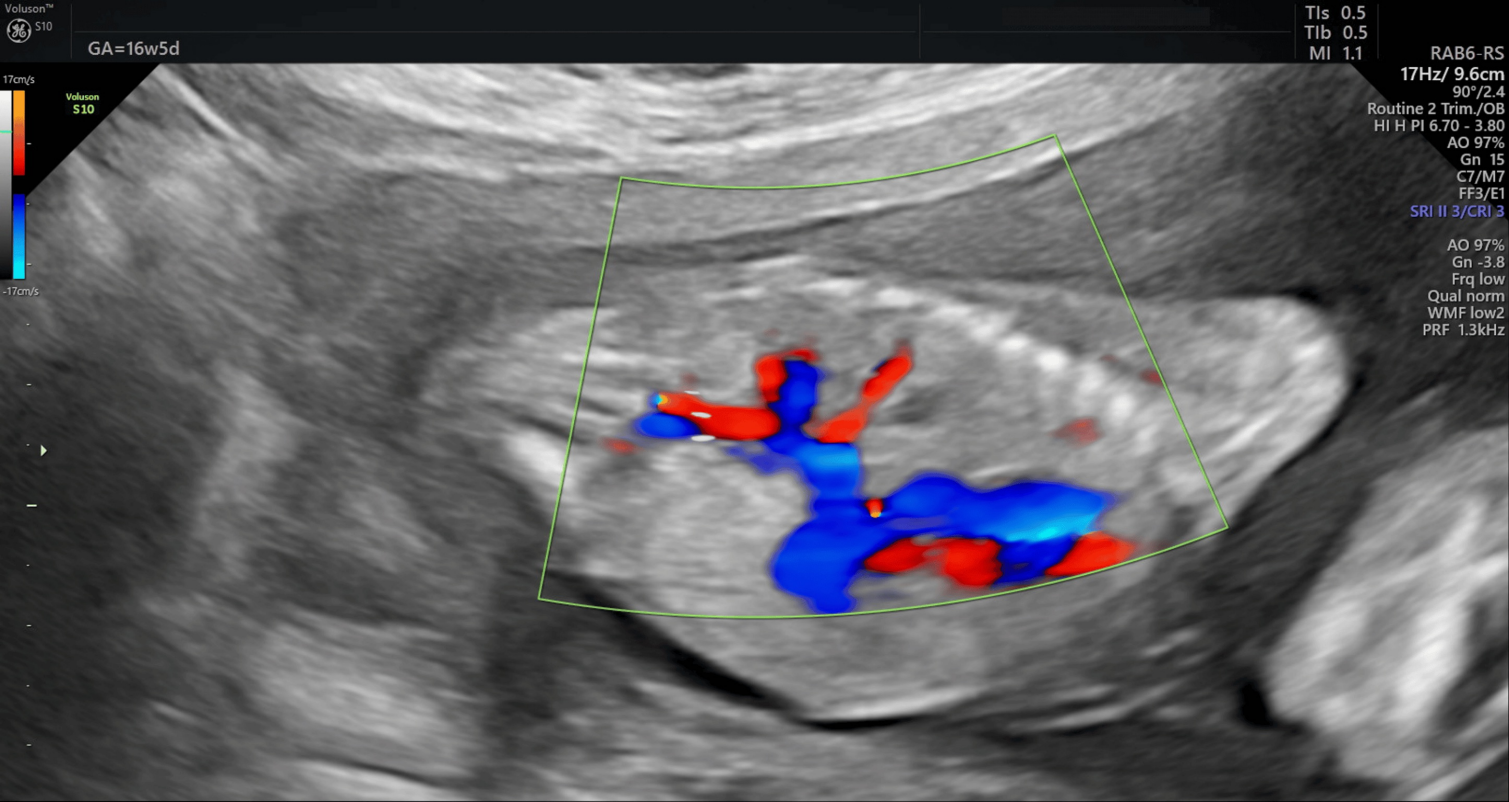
Doppler ultrasound image showing the normal blood supply of both fetal kidneys.
